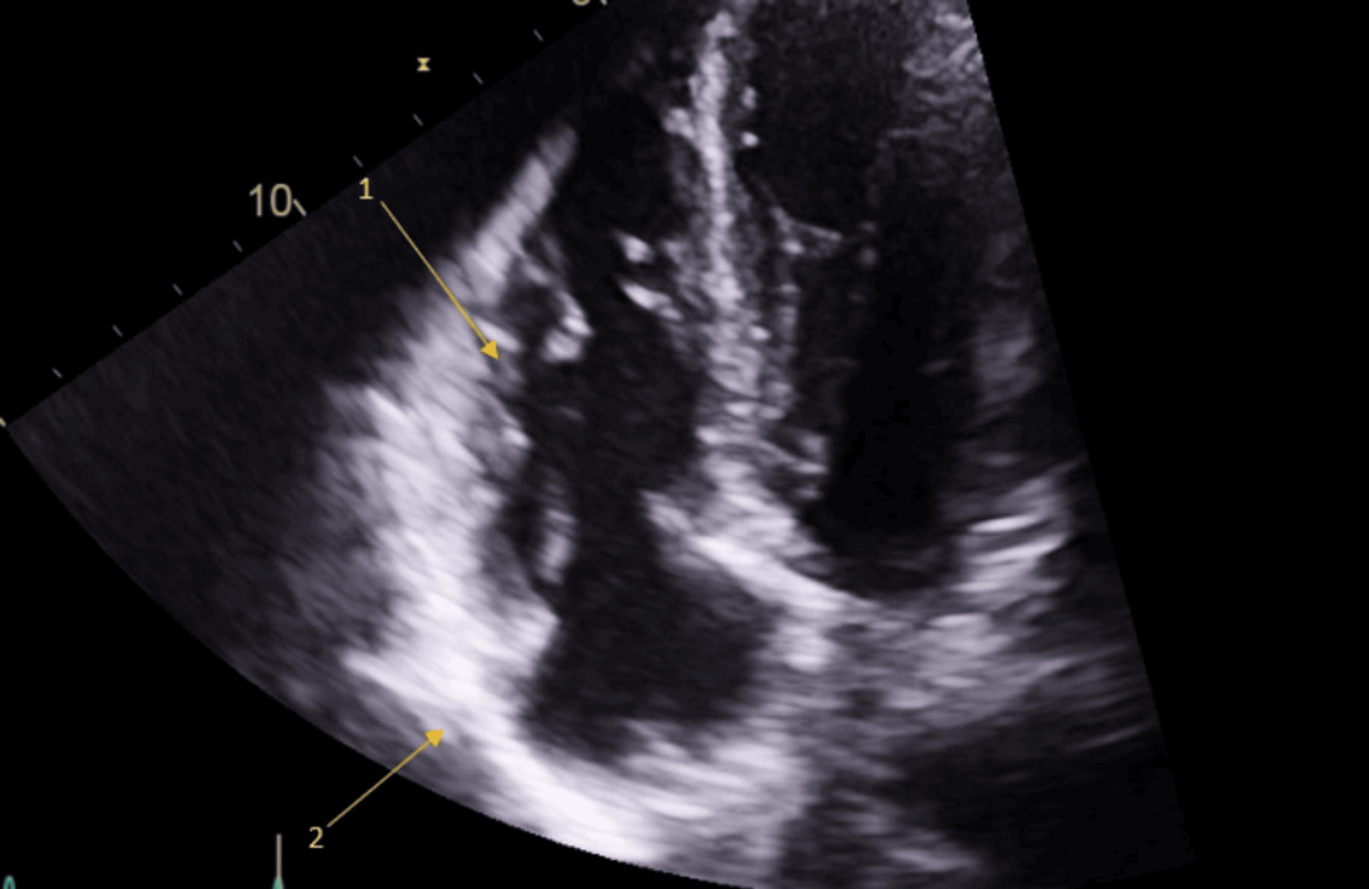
Transthoracic echo showing that the mid right ventricular wall appears aneurysmal and trabeculated Arrow 1: trabeculated and aneurysmal right ventricle; Arrow 2: brightening of pericardium
